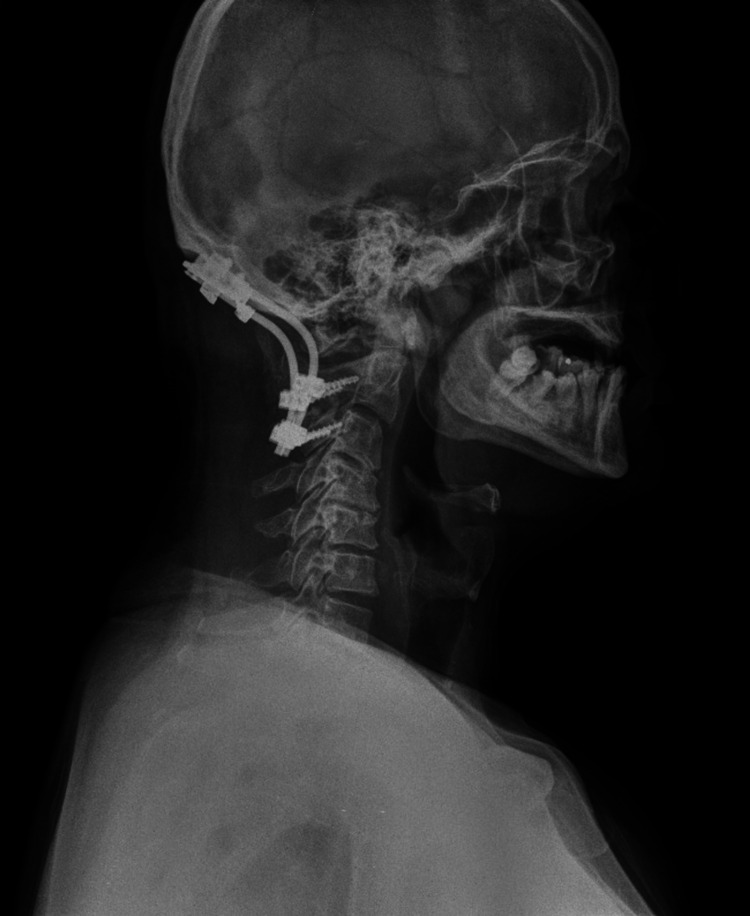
Profile X-ray of the cervical spine after occipitocervical fusion.
